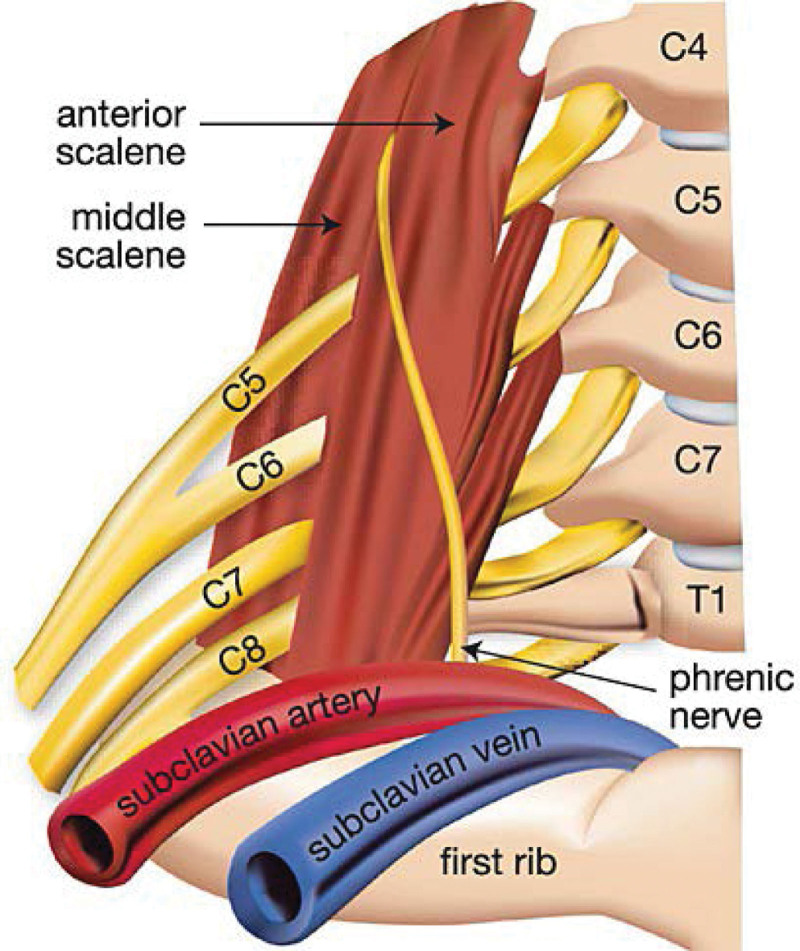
The usual pathway of the C5 and C6 nerve roots passing behind the anterior scalene muscle. Adapted from Flavel et al Sonography 2014;1(1):25–29, with permission.^[5]^ C5 = cervical 5, C6 = cervical 6.
